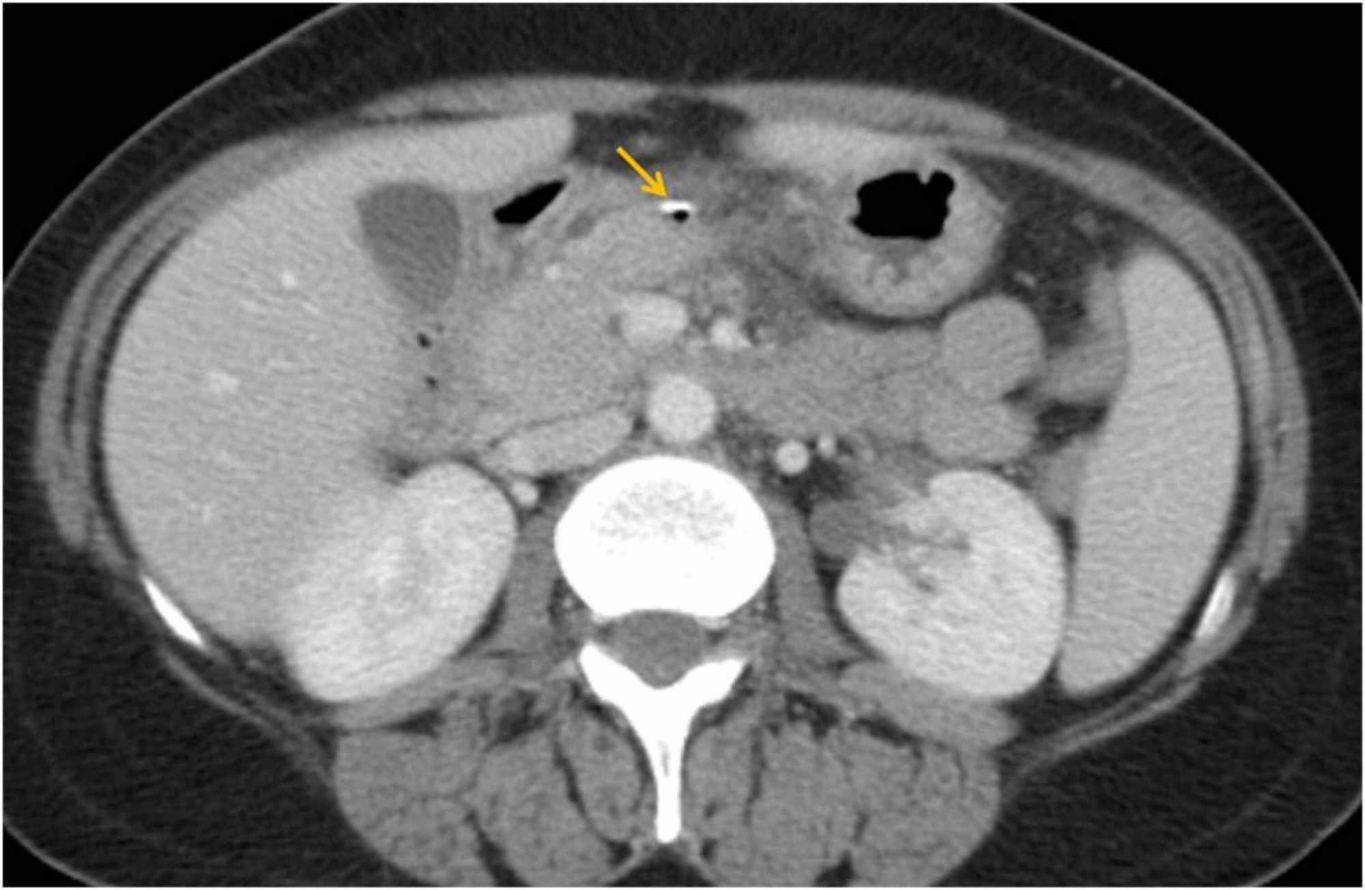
Abdominal CT: axial view The same patient in the axial view. The yellow arrow shows the fish bone located at the lesser omentum.
